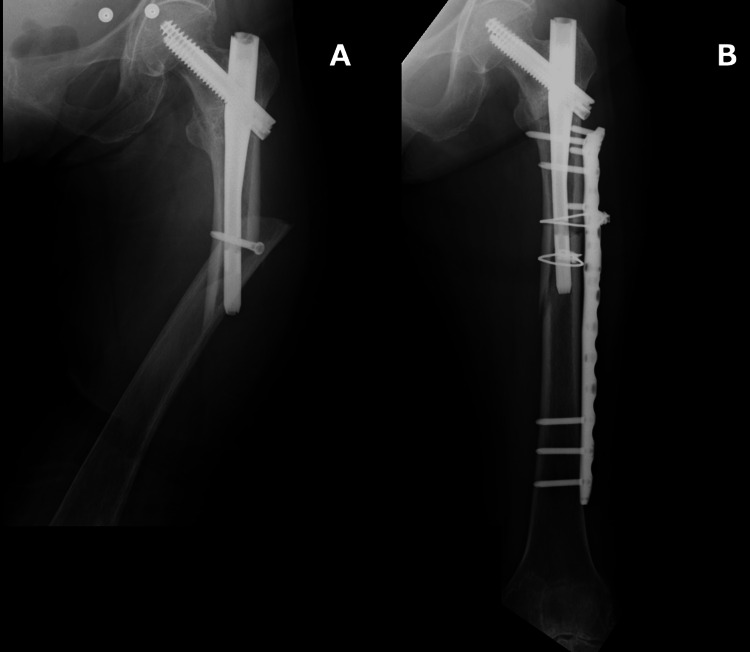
Radiograph findings A: preoperative anteroposterior radiograph; B: postoperative anteroposterior radiograph
